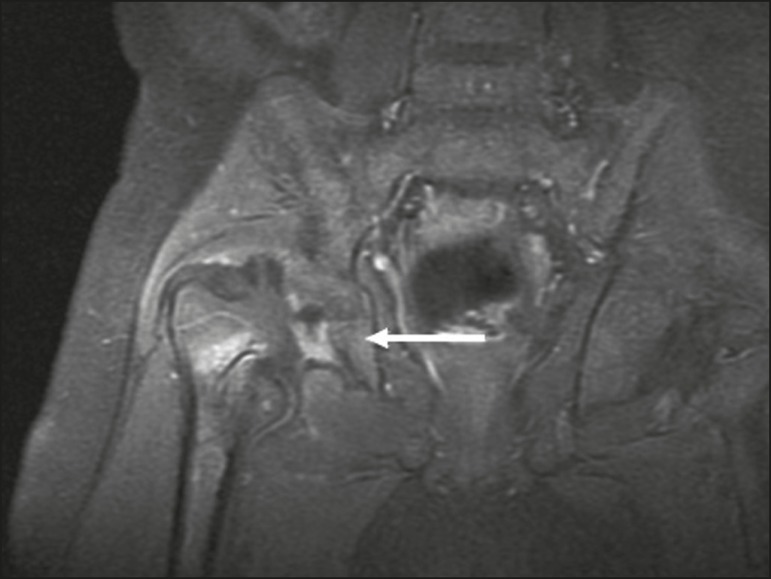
MRI of the hip. Contrast-enhanced, coronal, fat-saturated T1-weighted sequence showing complete destruction/resorption of the femoral head, accompanied by severe deformity of the femoral neck and acetabulum.
